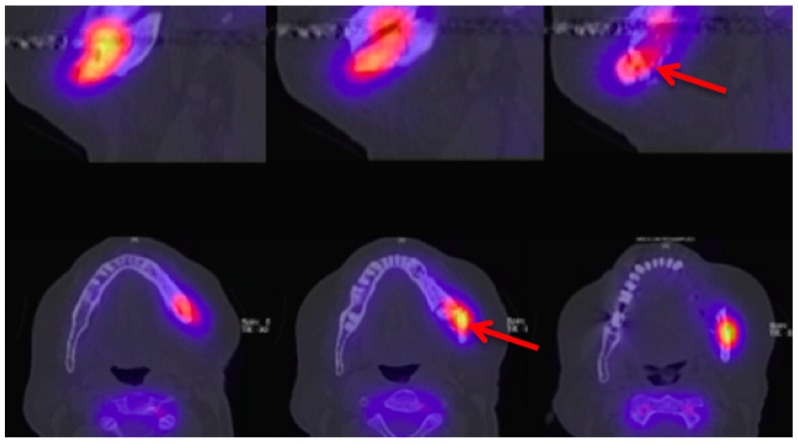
Technetium-99m-3,3-diphosphono-1,2-propanodicarboxylicacid (99Tcm-DPD) SPECT/CT (Siemens, Symbia) Patient: 66 years old, male, secondary osteoporosis due to castration, alendronate. First row sagittal, second row axial view; 4.5 h after injection (bone phase). The uptake in the left mandible is clearly visible (red arrow). For the panoramic radiograph see Figure 3. AAMOS staging: stage 3.
